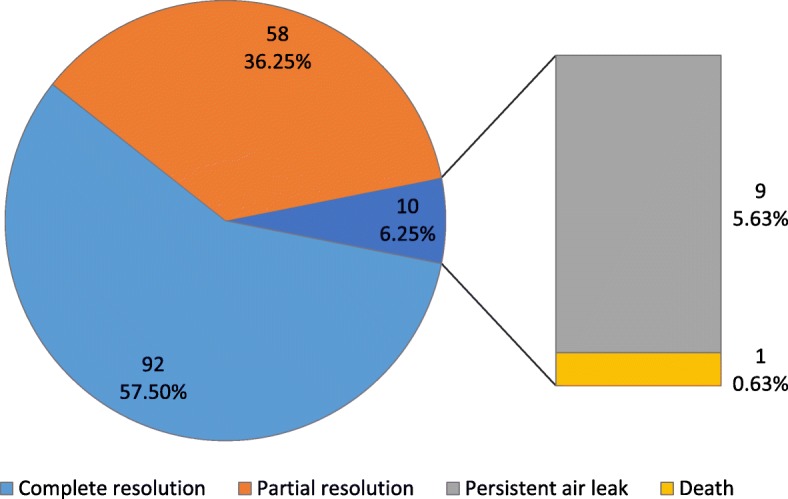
Outcome of radiograph resolution
